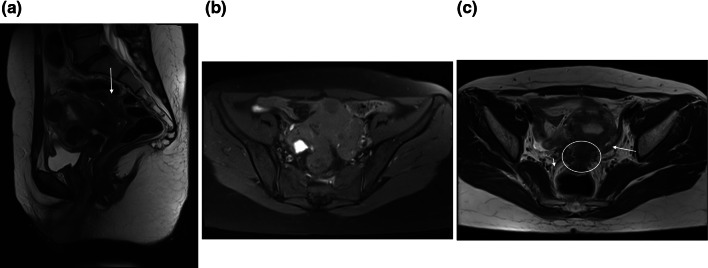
a T2 weighted sagittal MRI image showed large hyposignal plaque of DIE involving the rectum. b T1 weighted axial fat-suppressed image in the same patient shows hypersignal typical endometrioma in the right ovary. c T2 weighted axial MRI image showed obliteration of posterior cul-de-sac, DIE in retrocervical and rectal wall, uterosacral (small arrow) and round ligaments (large arrow) with adhesions
